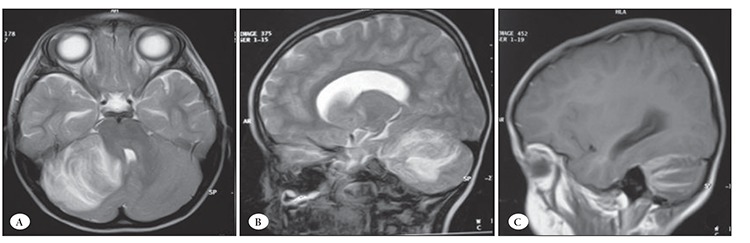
Axial (A) and sagittal (B-C) T1- and T2-weighted MRI with Gd-DTPA show the linear heterogeneously enhanced mass inthe right cerebellar hemisphere.
